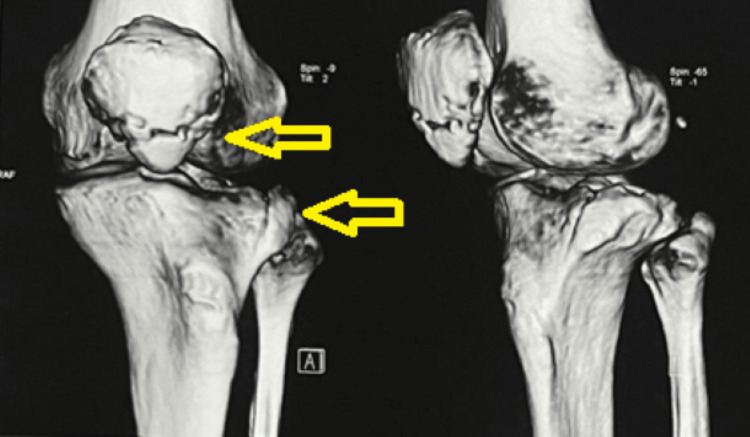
The 3D CT scan of the patella and tibia showing fractures marked with yellow arrows
